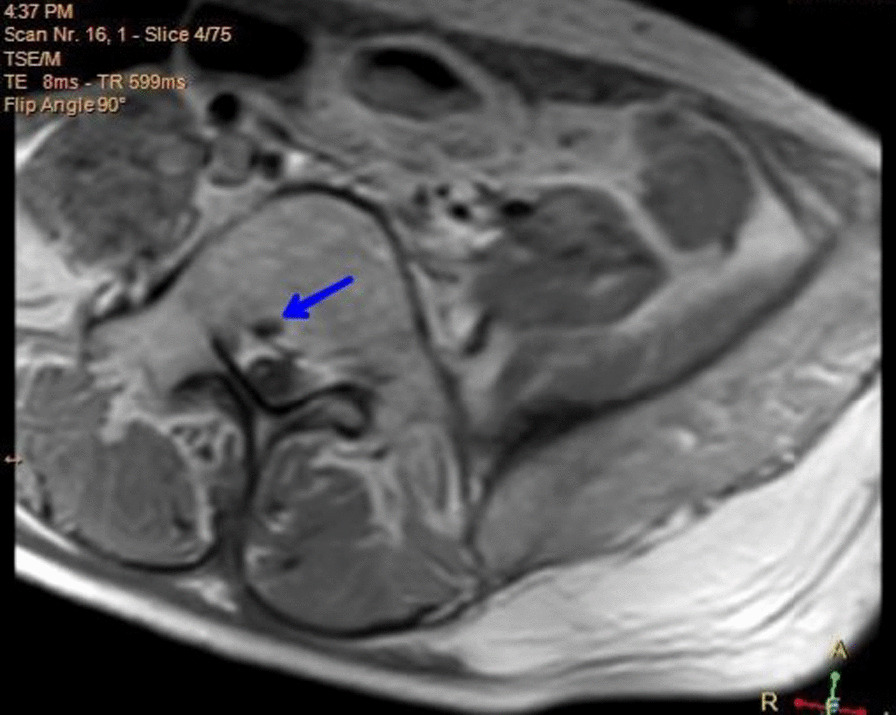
T1-weighted postcontrast MRI transversal view showing metastasis (blue arrow) to the second lumber vertebra
